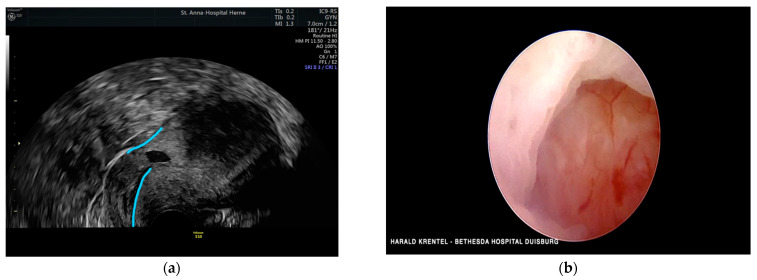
(a) Transvaginal ultrasound showing a uterine niche; (b) hysteroscopic view of uterine niche.
